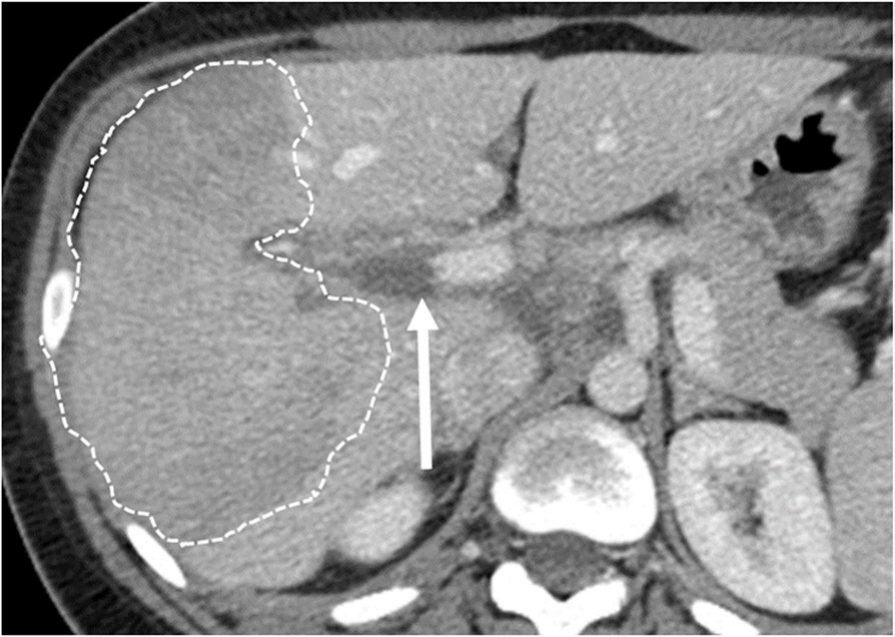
Pre-eclampsia (PE) outcomes in a 24-year-old primiparous woman. This patient did not perform imaging studies during pregnancy, but presented with persistent right upper quadrant pain around delivery, increased AST/ALT and arterial blood hypertension; hence, PE was suspected. Axial, portal venous phase CT scan performed after childbirth demonstrates portal vein thrombosis (arrow) and parenchymal infarction (dotted lines)
